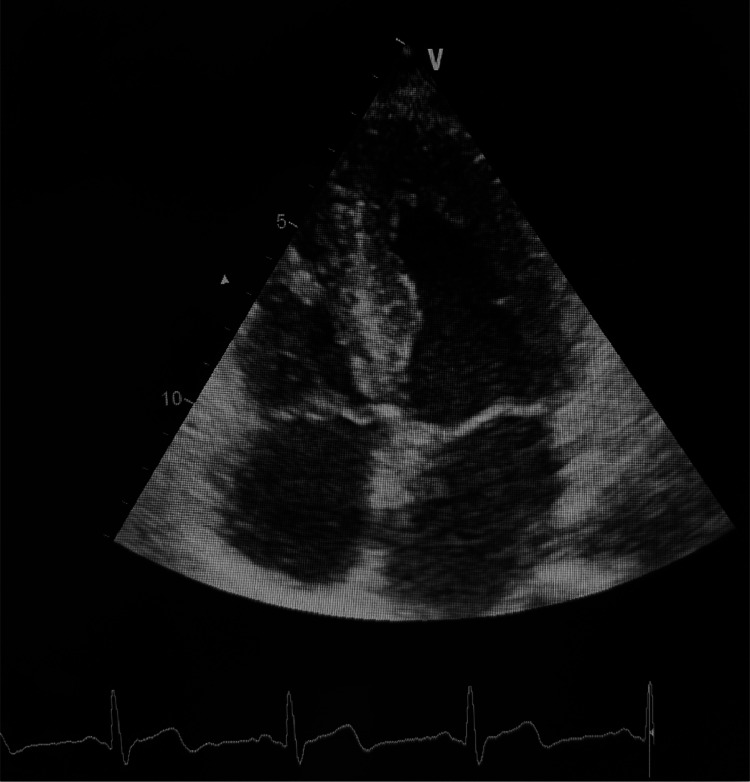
Three month follow up trans thoracic echocardiographic images of apical 4 chamber demonstrating clear resolution of the intra cardiac clot of the right atrium.
